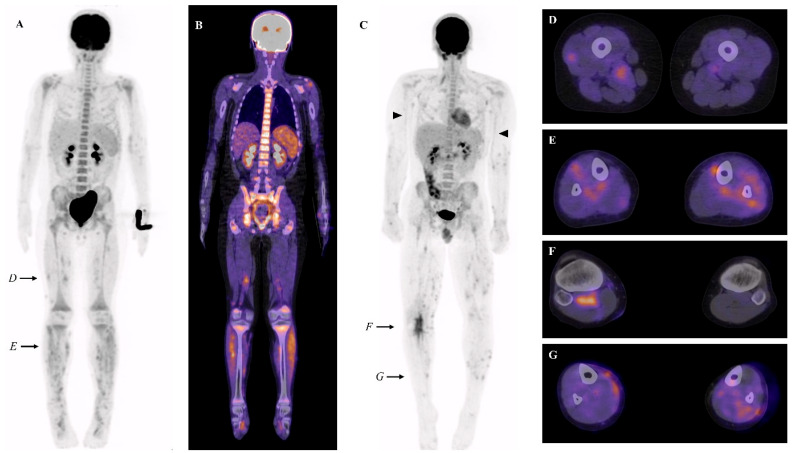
(A,B) Maximal intensity projection (MIP) (A) and representative fused FDG PET-CT coronal view (B) of patient 1, showing diffuse hypermetabolic foci in the lower limbs. (C) MIP FDG PET-CT of patient 2, showing diffuse hypermetabolic foci in the lower limbs, vascular uptake in both brachial arteries (black arrowhead), and the right popliteal region. (D–G) Fused FDG PET-CT transverse views showing foci of increased muscular and subcutaneous FDG uptake in patients 1 and 2 (levels of the cross-sectional views are shown by black arrows in (A,C)).
